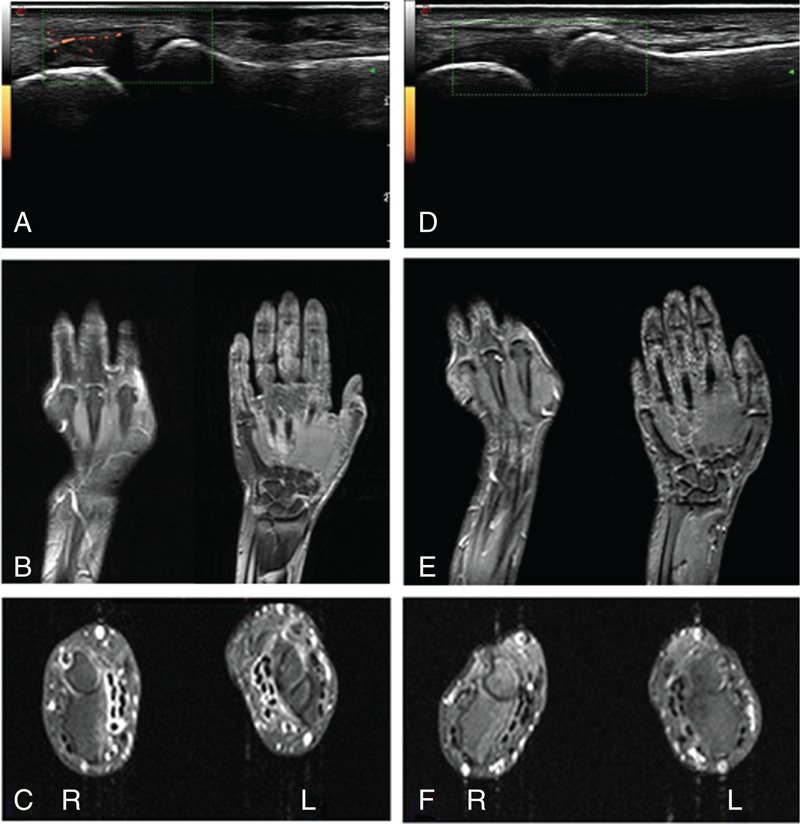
Ultrasonography images of second MCP joint of the left hand and fat-sat gadolinium-enhanced T1-weighted Turbo Spin Echo coronal and transverse magnetic resonance imaging images of left hand and wrists at baseline (A–C) and after periodontal disease treatment (D–F). (A) Moderate active synovitis of the II MCP joint of the left hand (power-doppler grade II). (B) Microerosions of second and third metacarpal head and inflammatory involvement of digital synovial sheaths of third and fourth finger and recessus ulnaris (prestyloideus). (C) Diffuse thickening (enhancement) of sheath of superficial and deep digital flexor tendon and extensor carpi ulnaris tendon of right wrist; less thickening of left wrist. (D) Second MCP joint of the left hand: absence of synovitis (power-doppler negative). (E) Marked reduction of synovial thickening of recessus ulnaris (prestyloideus) and the synovial sheath of third and fourth finger of flexor tendon; persistence of the minimal erosion of third metacarpal head (contrast enhancement). (F) Remarkable reduction of right flexor tendon and extensor carpi ulnaris tendon sheaths thickening. MCP = metacarpophalangeal.
